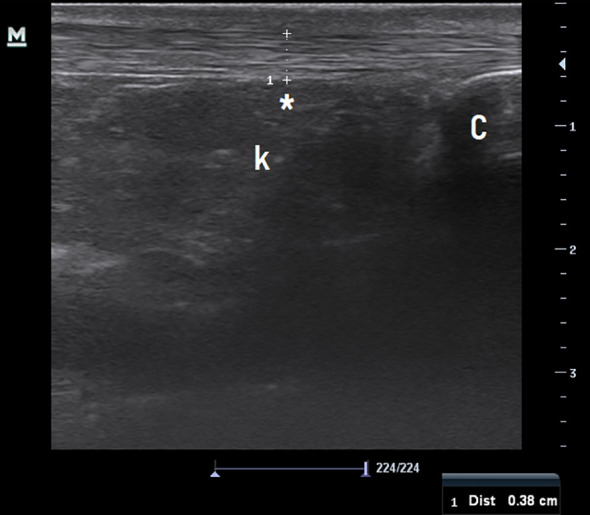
Ultrasound measurement of the Achilles tendon in a normal-weight participant (control group). Asterisk: Achilles tendon thickness at the midportion; C: Calcaneus. K: Kager’s fat pad.
